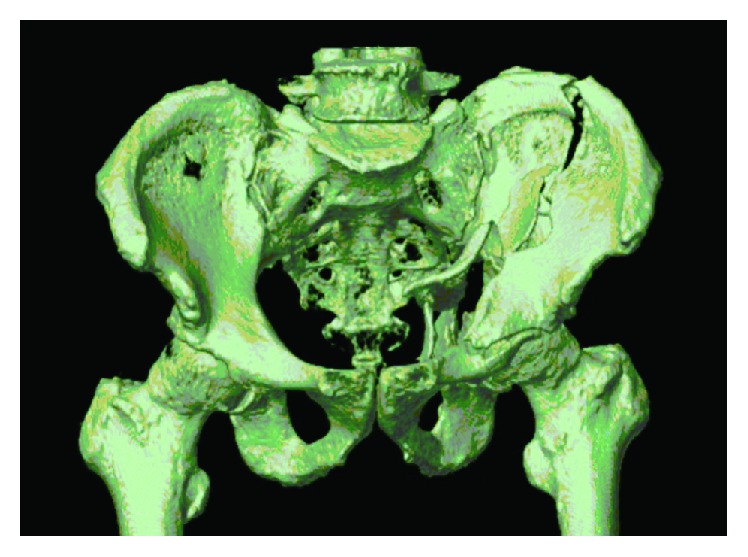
Three-dimensional computed tomography scan of the fracture showing displacement of both columns and impaction of the femoral head.
